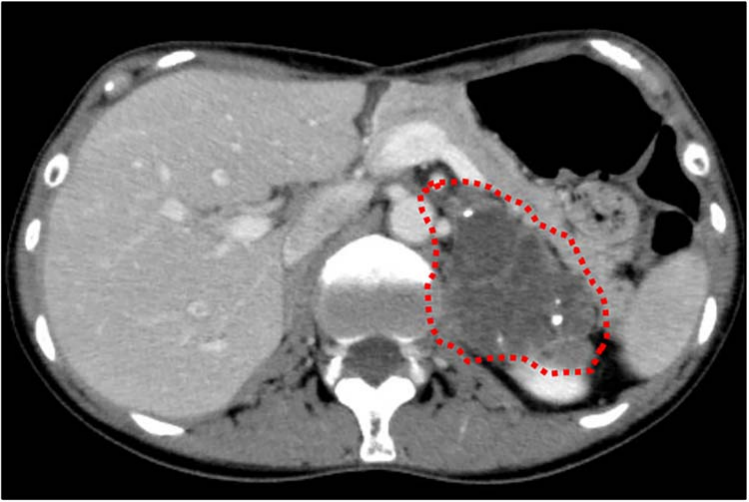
Abdominal CT findings. A calcified tumour with a maximum diameter of 6.0 cm was observed in the left adrenal gland.
